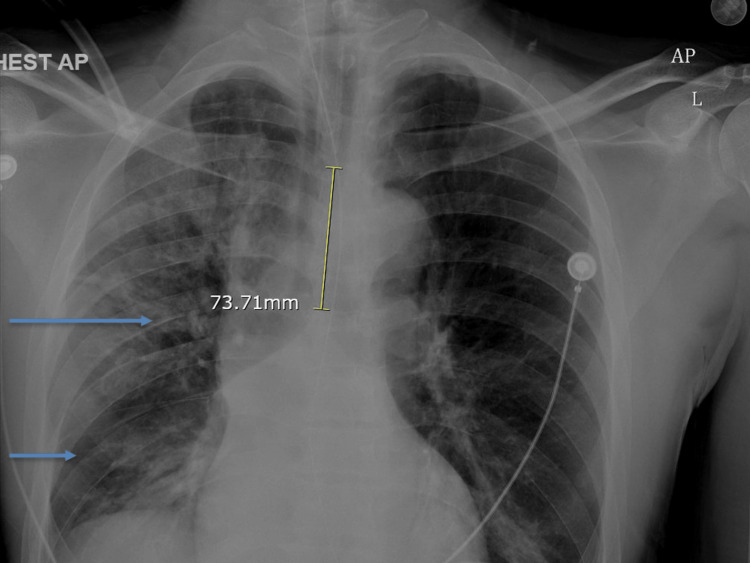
Chest X-ray showing airspace opacities in the right mid and lower lung (arrows)
